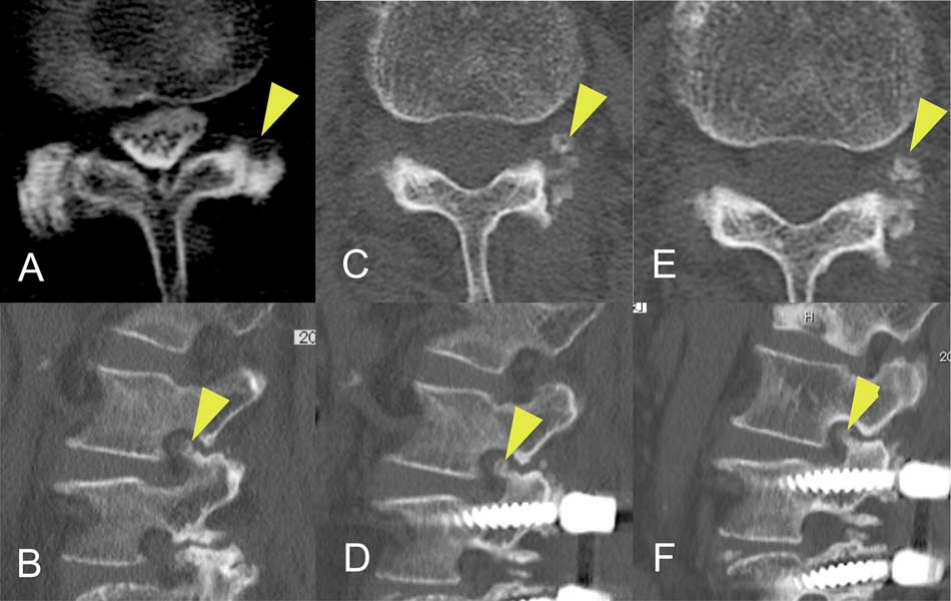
Progression of ossification of the ligamentum flavum (OLF) on computed tomography (CT) images obtained before onset of radiculopathy. (A) OLF was not found on a CT scan obtained 8 years earlier. (B) A CT image obtained 20 months earlier shows a small isolated area of OLF in the foramen. (C, D) A CT image acquired 4 months earlier shows that the area of OLF had grown slightly and was integrated with the superior articular process. (E, F) A CT image obtained at the time of surgery shows further expansion of OLF.
